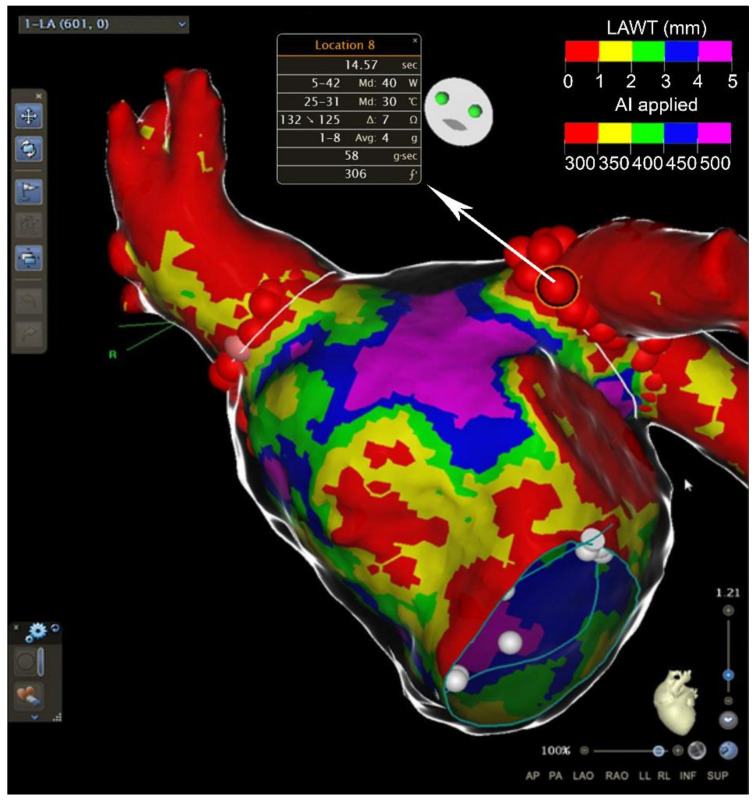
Usefulness of cardiac CT in atrial fibrillation ablation. Anterior view of the left atrium after atrial fibrillation ablation around the pulmonary veins. Atrial wall thickness (in mm) is depicted with different colors on the map. Major values in radiofrequency applications (AI) are used in thicker areas. AI = ablation index. LAWT = left atrial wall thickness.
